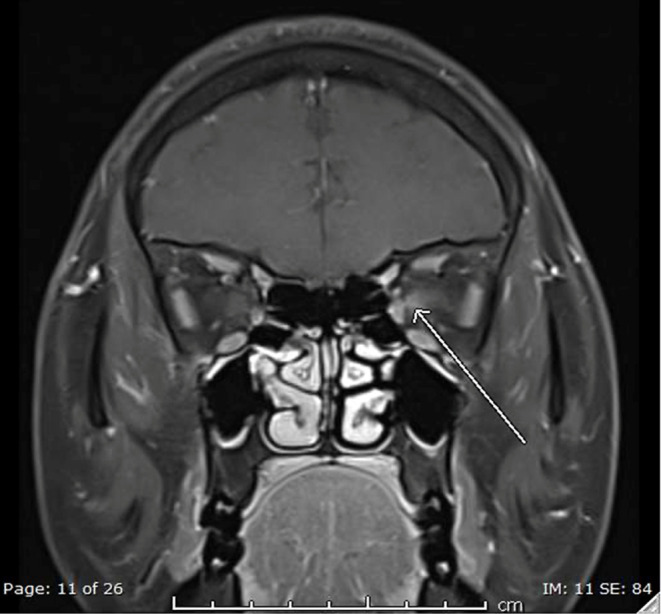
T1-Weighted Coronal Section MRI with Contrast. Contrast-enhancement in the medial intracanalicular part of the left-sided optic nerve sheath (arrow).
